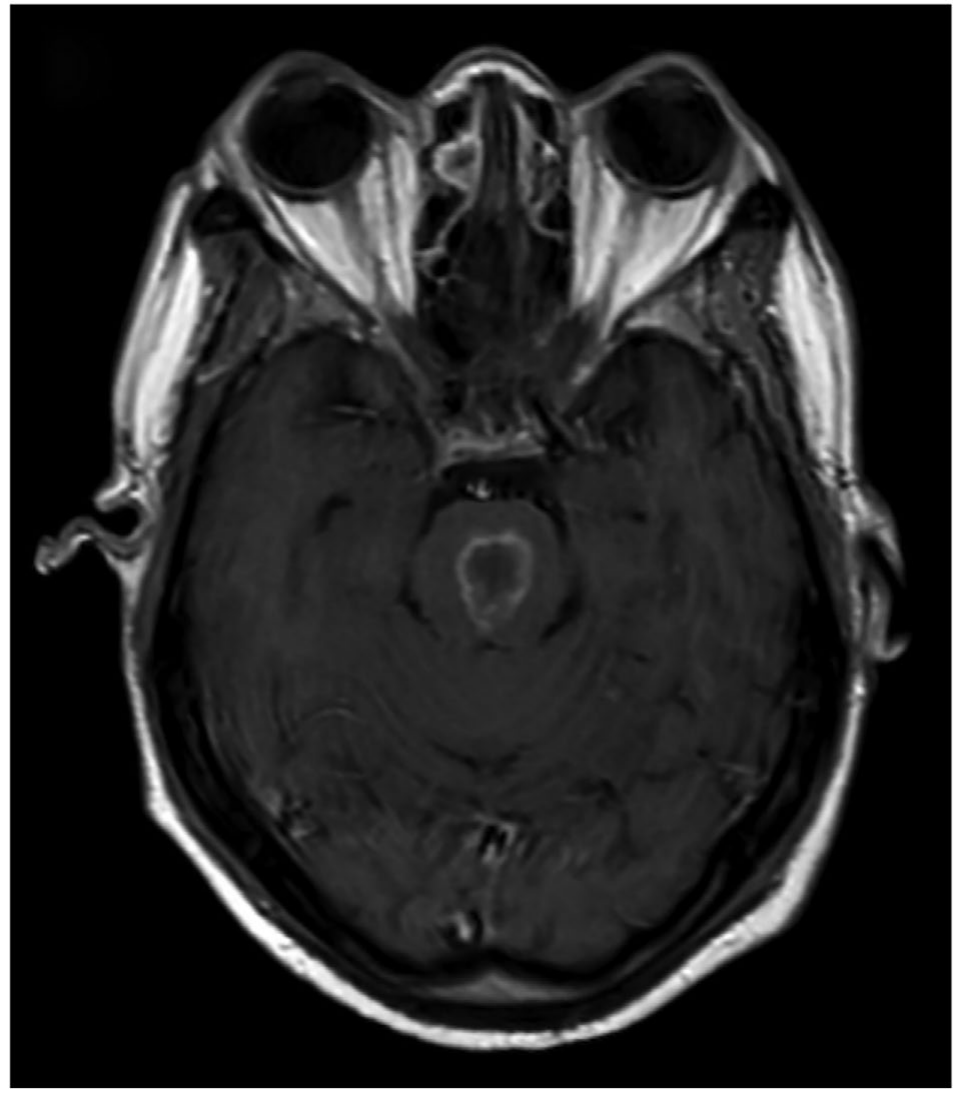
Axial, gadolinium‐enhanced, T1‐weighted MRI image showing abscessual evolution of a brain lesion in the pons 9 days after the onset of neurological symptoms
